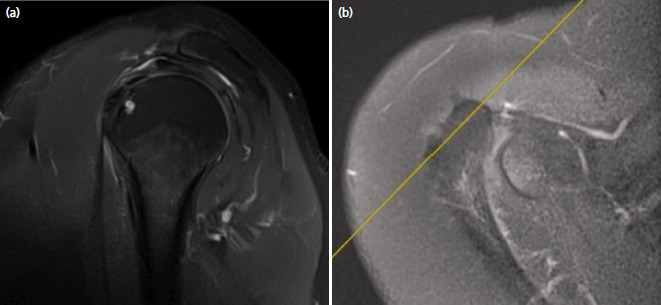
(a) Parasagittal magnetic resonance image on T2-weighted fat-suppressed sequences on which observers made evaluations. (b) Selection of adequate slice position on an axial localising image.
